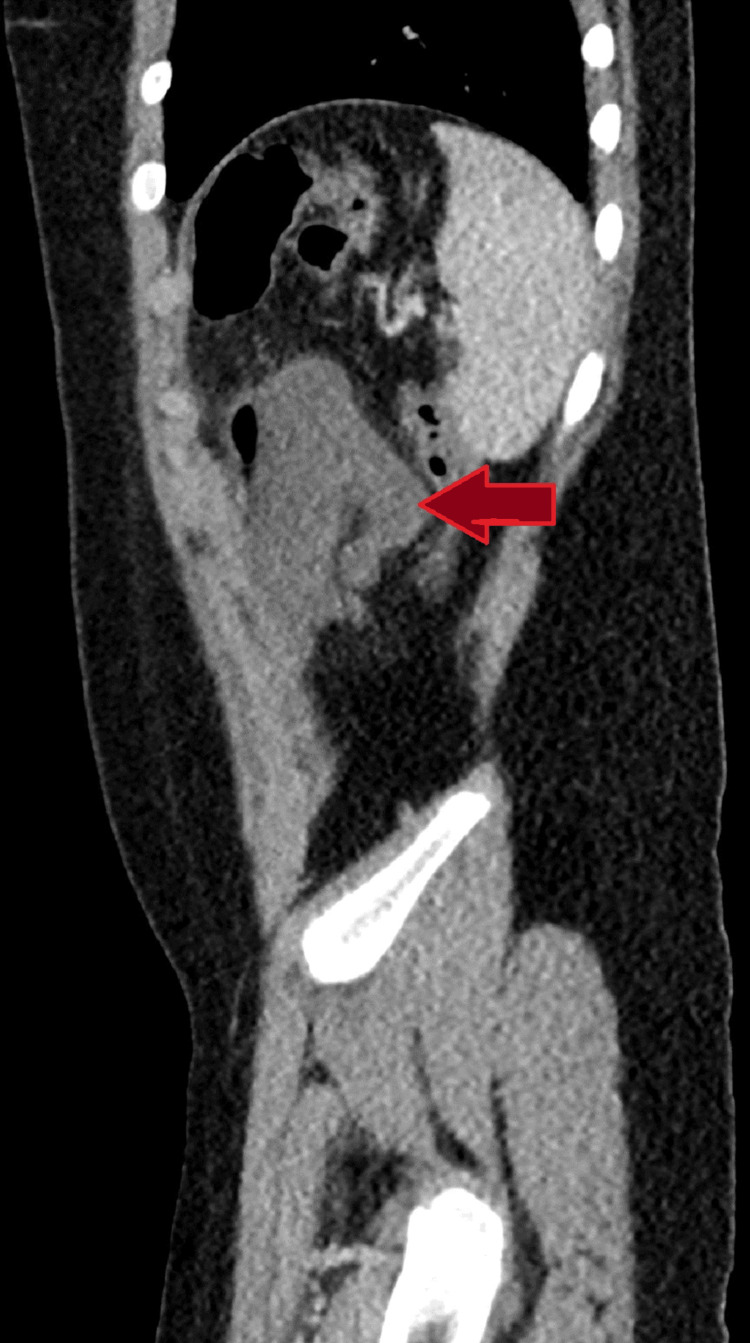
CT Scan - Abdomen Sagittal plane of CT demonstrates dilatation of a small intestinal loop in the central upper abdomen measuring about 28 mm in diameter (red arrow).
